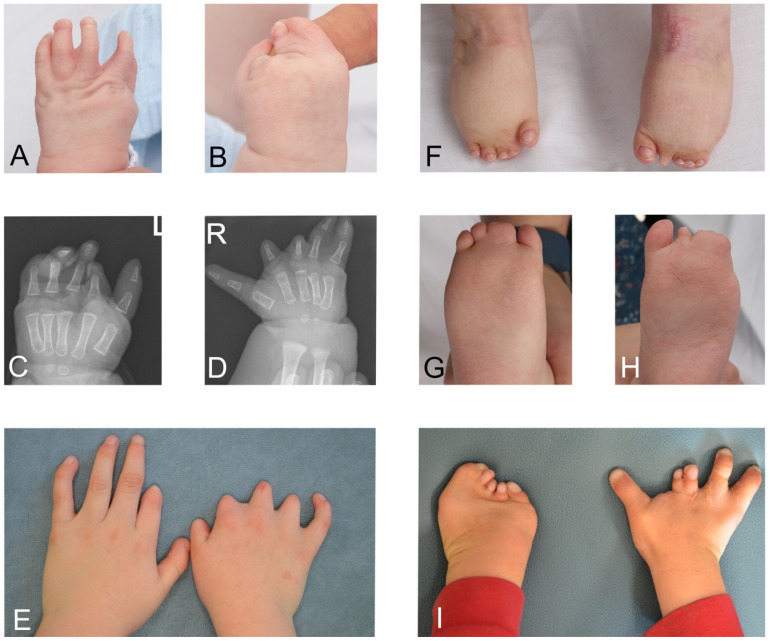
Limb anomalies in Moebius syndrome. (A–D) Photo and radiographs of right and left hands of patient with bilateral symbrachydactyly. (E) Bilateral symbrachydactyly. (F–H) Hypoplastic toes, talipes and syndactyly of feet. (I) Bilateral complicated symbrachydactyly.
